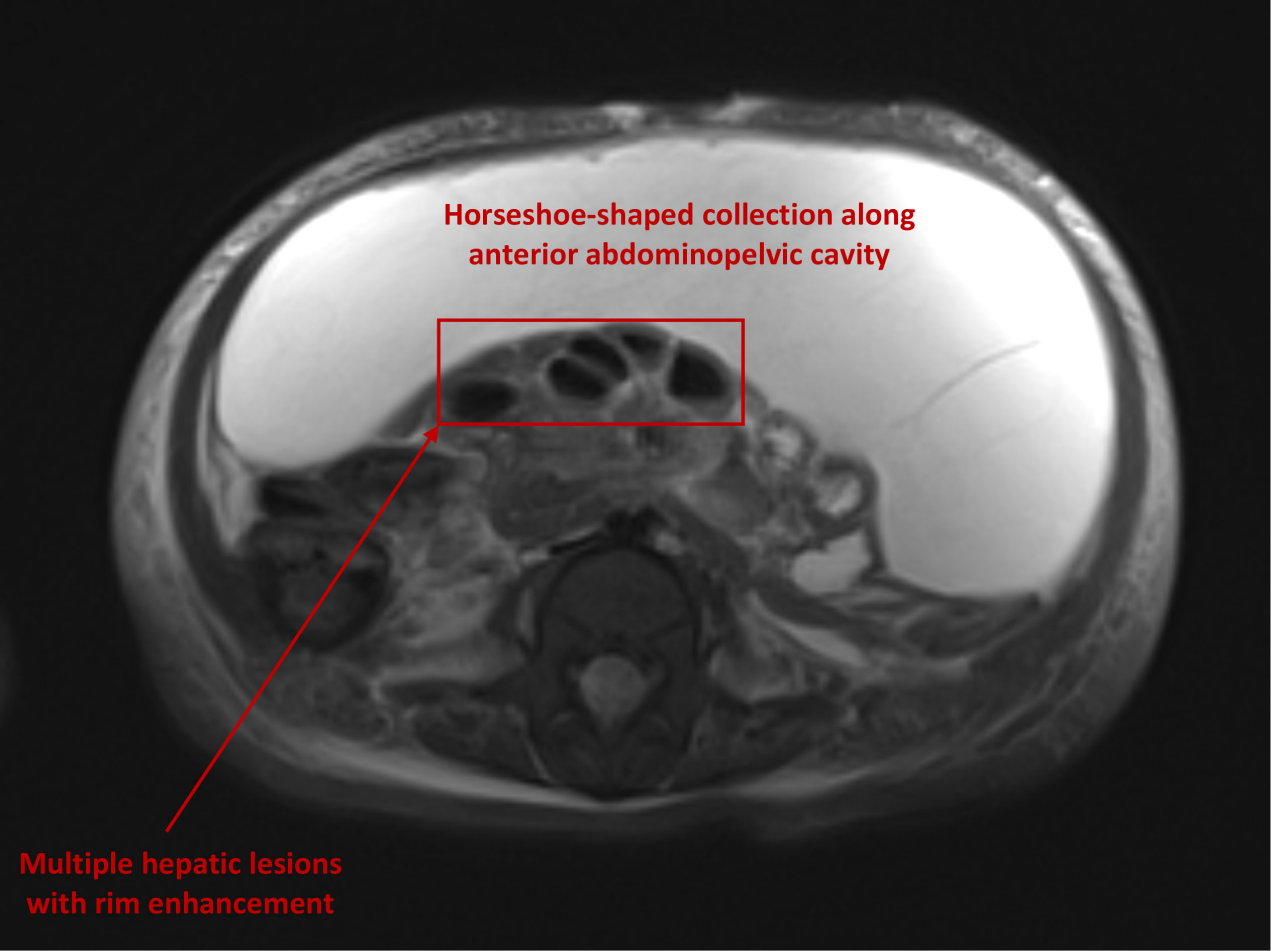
Liver abscesses and ascites on abdominal MRI.
